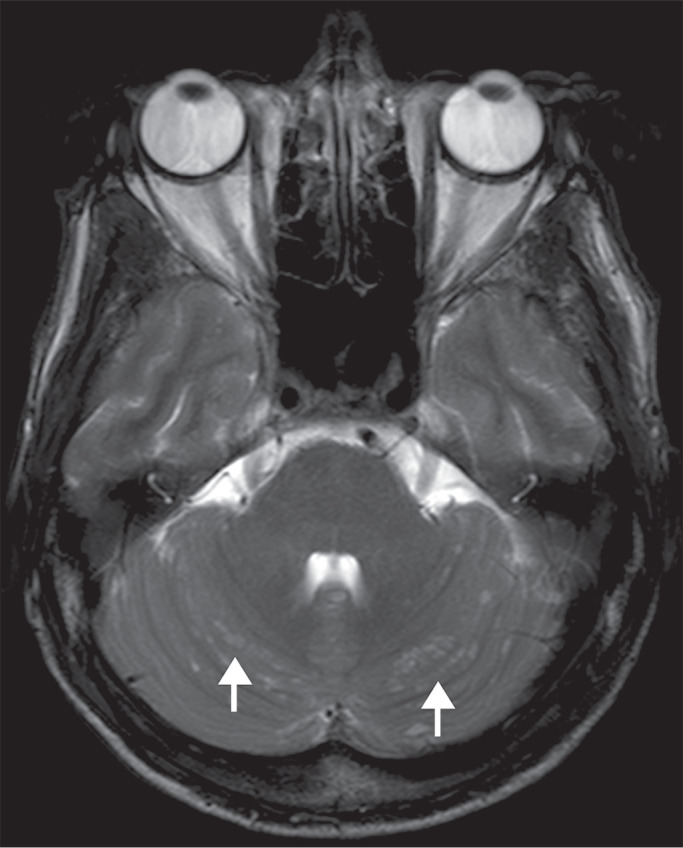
Axial T2-weighted image showing multiple hyperintense ovoid lesions in the cerebellar hemispheres (arrows). Those lesions had demonstrated hypointense signal on T1-weighted images, with punctate enhancement on after contrast administration, without calcifications or bleeding foci and no restricted diffusion (images not shown). The final diagnosis was CNS cryptococcosis.
